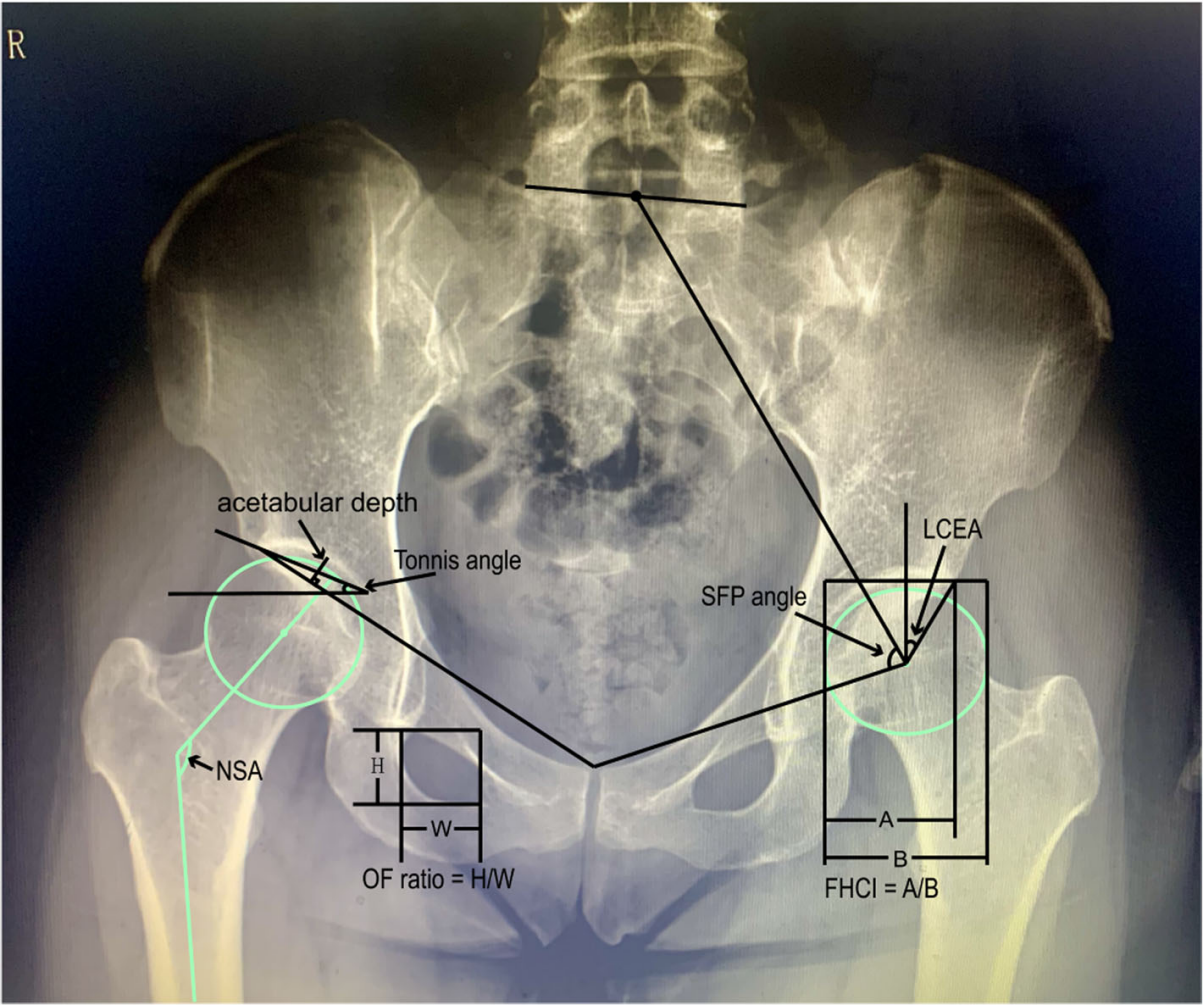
Parameter measurement in anteroposterior pelvic radiographs. NSA, neck-shaft angle; LCEA, lateral center edge angle; FHCI, femoral head coverage index; SFP angle, Sacro-femoral-pubic angle; OF ratio, obturator foramen ratio
